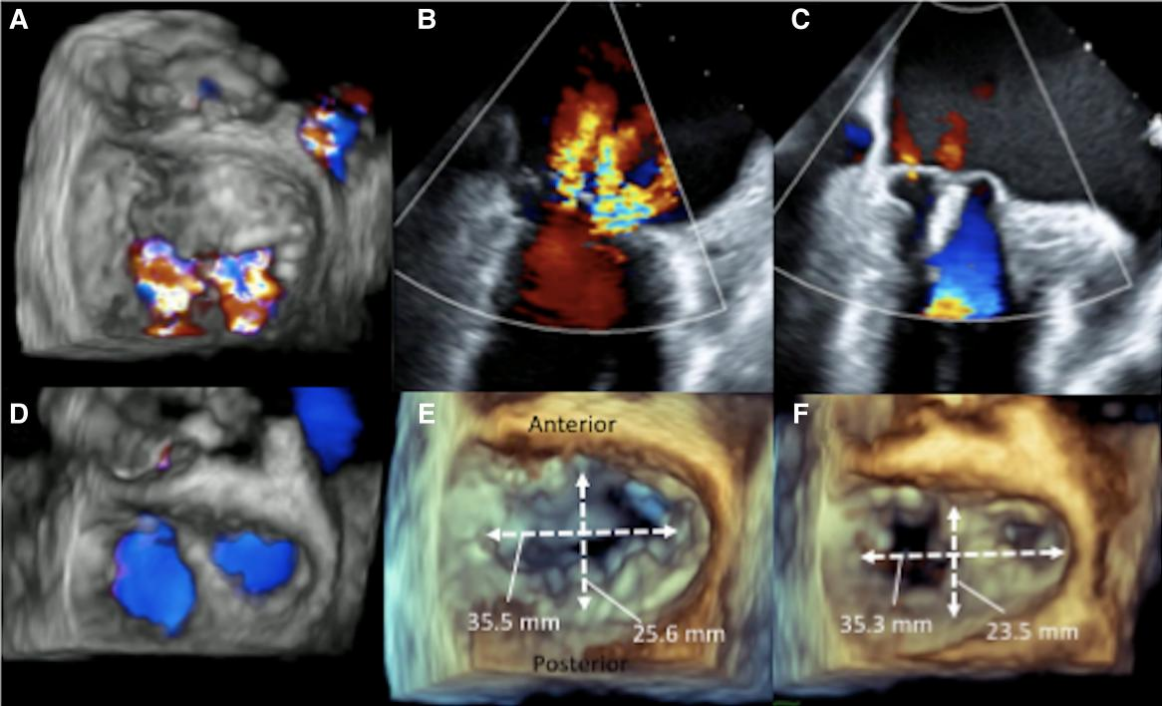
Transoesophageal echocardiography of the mitral annuloplasty effect after MitraClip implantation. (*A*) and (*B*) Two separated MR jets, including a central-medial jet and a lateral jet. (*C*) Significant reduction of all MR jets after a clip deployment. (*D*) NTW clip deployment in the 11–5 5 o’clock direction. (*E*) The mitral annular shape before MitraClip implantation. (*F*) Change of the mitral annular shape after MitraClip implantation with the reduction of the anterior–posterior diameter. MR, mitral regurgitation.
